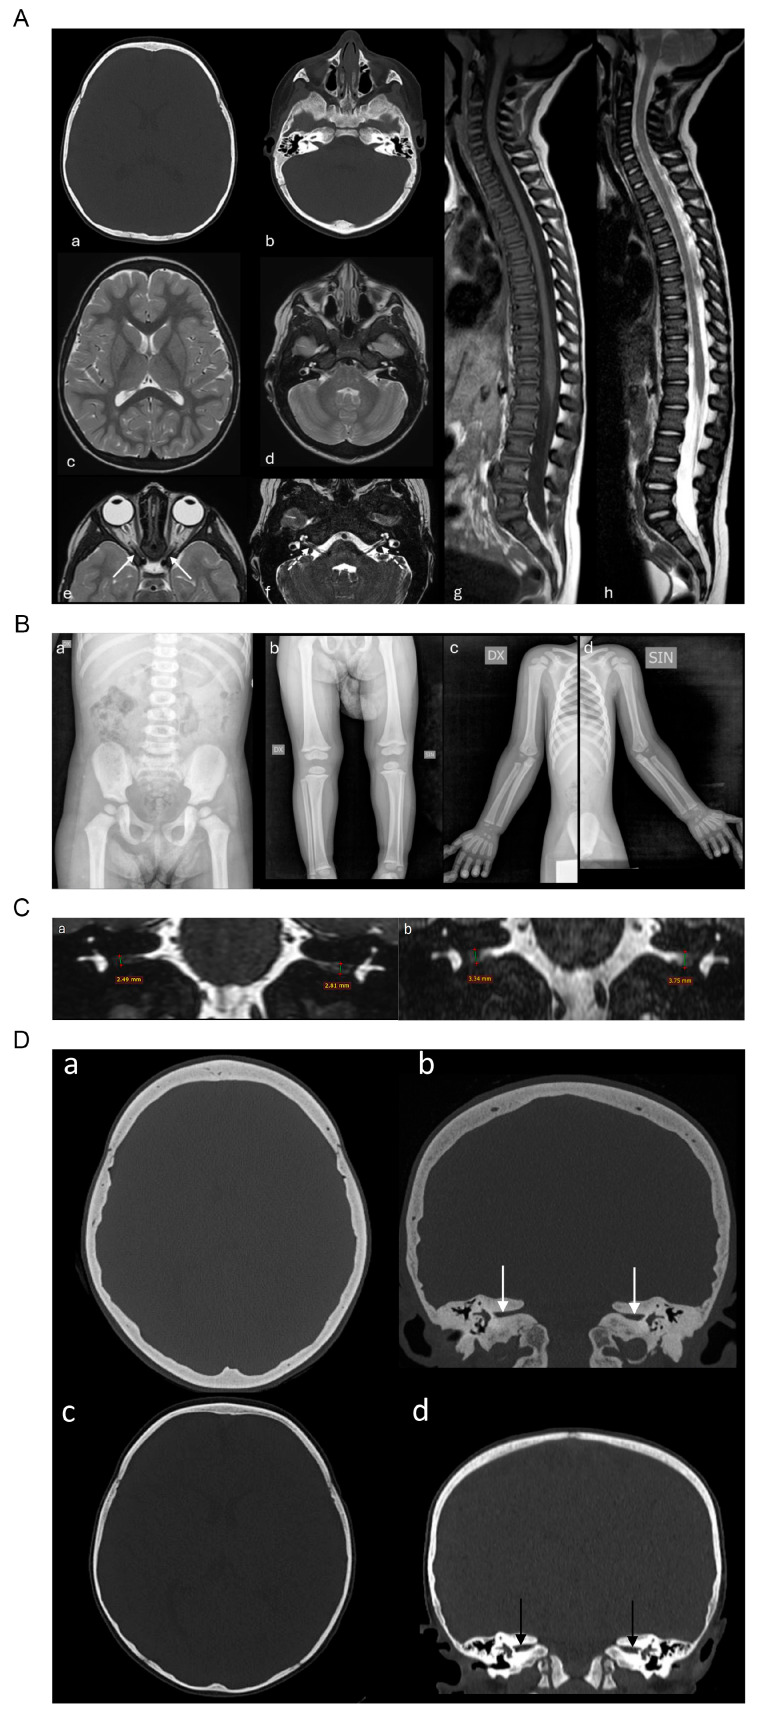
(A). Brain CT (a,b) and MRI (c,d) show sclerosis and hyperostosis with thickening of the cranial theca and basicranium. Arrows indicate the reduction in the caliber of optic foramina and internal acoustic canals (e,f). Spine MRI (g,h) reveals diffuse and mild sclerosis of vertebra both in T1W and T2W. (B). X-ray bone survey shows generalized increase in bone density with diffuse cortical thickening, showed in different sections (a,b,c,d). (C) Five-year follow-up coronal MRI with 3D-DRIVE sequences shows severe stenosis of internal acoustic canals (b) compared to baseline MRI (a). (D). Five-year follow-up CT (a,b) compared to baseline study (c,d) demonstrates progressive increase of hyperostosis and thickening of the cranial theca and temporal bones with severe stenosis of internal acoustic canals (arrows).
